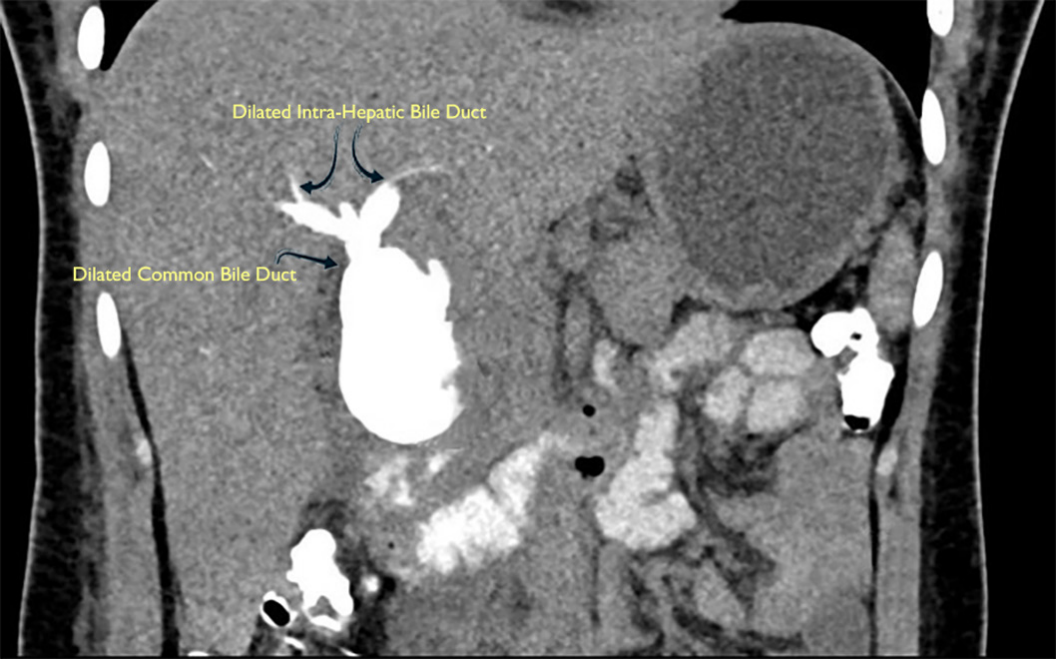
CT cholangiogram showing fusiform dilatation of the entire common bile duct (single arrow), with intrahepatic bile duct dilatation (two arrows).
